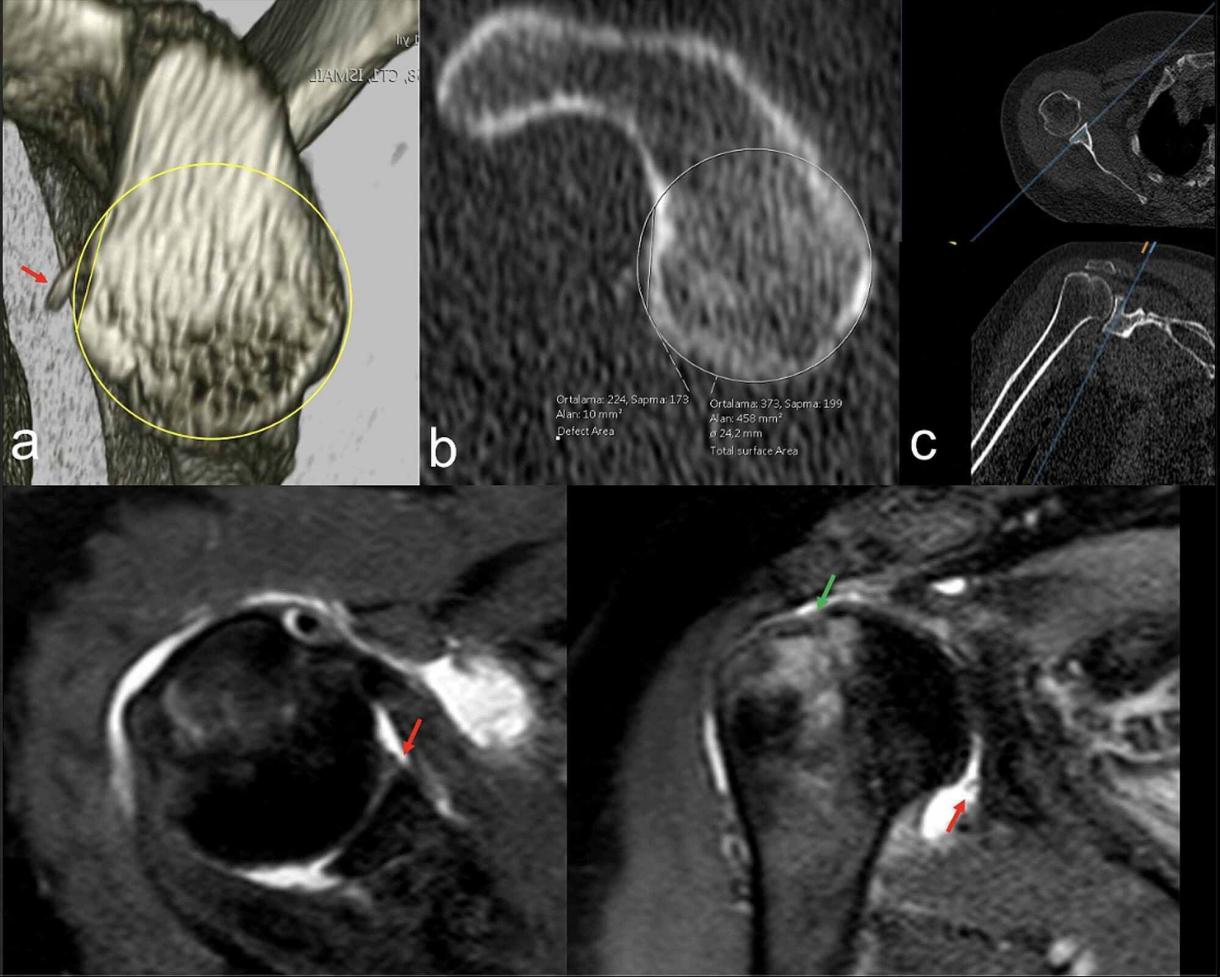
Preoperative images of case 1 (a) Three-dimensional CT image of glenoid; (b) measurement of the bone Bankart defect; (c) MPR images; (d) axial MRI showing defective labrum; (e) Hill-Sachs defect (green arrow) and labral defect (red arrow). CT: computed tomography; MRI: magnetic resonance imaging; MPR: multiplanar reformation.
